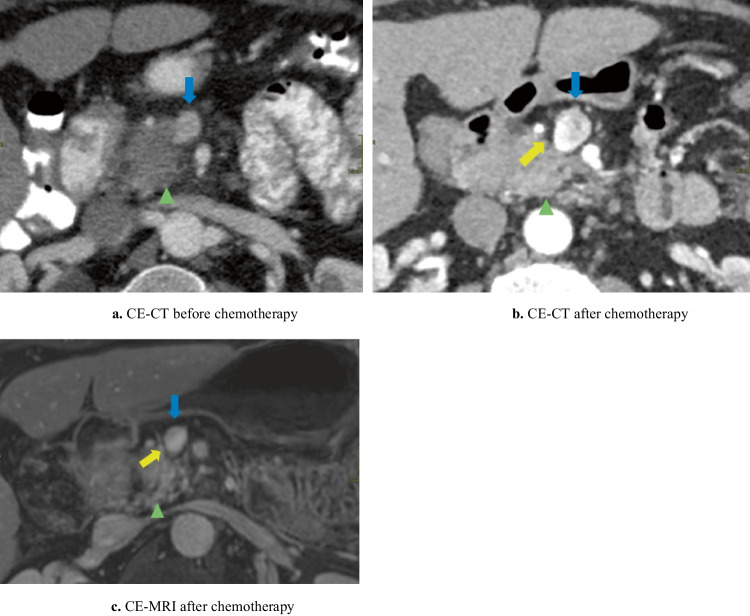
Halo sign on CE-MRI and CE-CT
